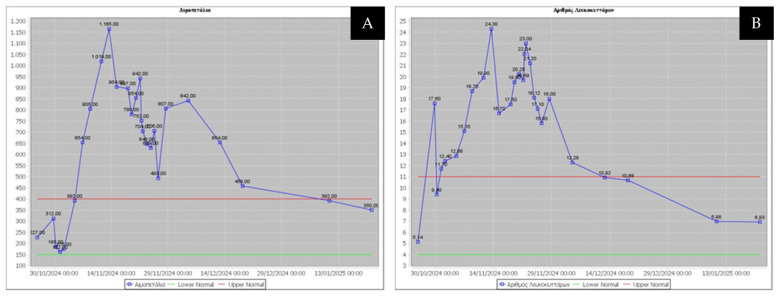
Thrombocytosis (A) and leukocytosis (B) were observed with a steep increase after the second postoperative week, indicating a developing infection that was probably related to the thoracic procedure. A thorough physical examination, cultures, and CT imaging are mandatory to reveal the source of infection. For patients receiving systemic chemotherapy, these findings alert us to oncoming sepsis. Once the source is recognized, surgical exploration should be encouraged. One week after the thoracoplasty procedure, there was a steep decline in inflammatory cell counts.
